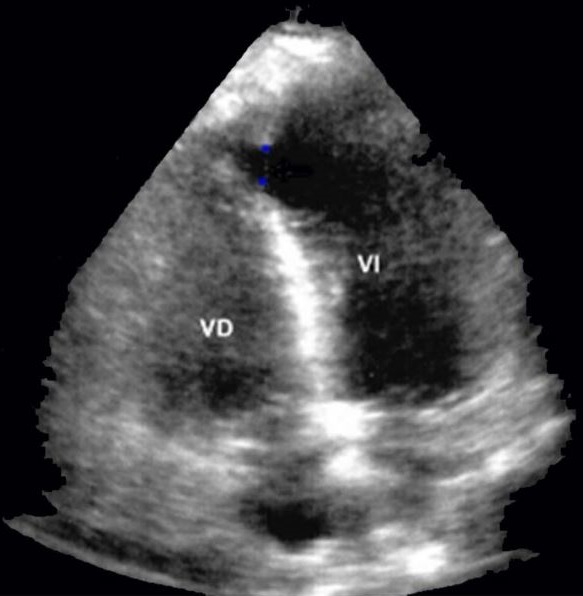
Coupe apicale quatre cavités d’echocardiographie montrant une rupture septale apicale
